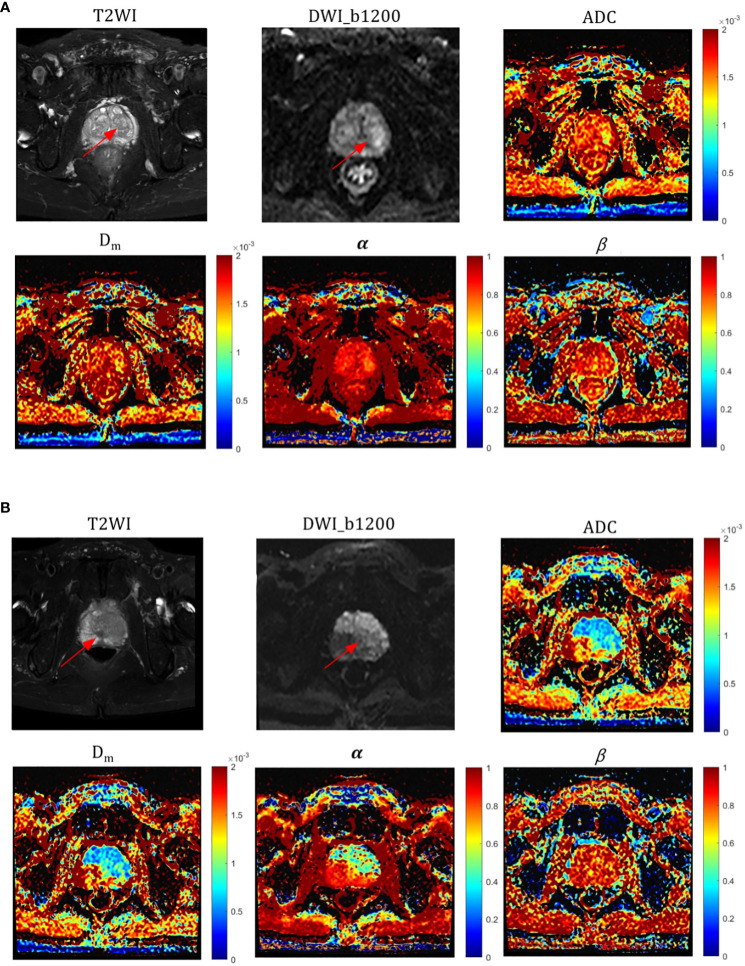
Representative images of T_2_WI, DWI, ADC, and CTRW parameters (red arrows represent lesions); (A) a patient with a low-grade (GG=2) pCa lesion and (B) a patient with a high-grade (GG=5) pCa lesion. ADC, α, and Dm values were significantly different in both high and low grade pCa.
